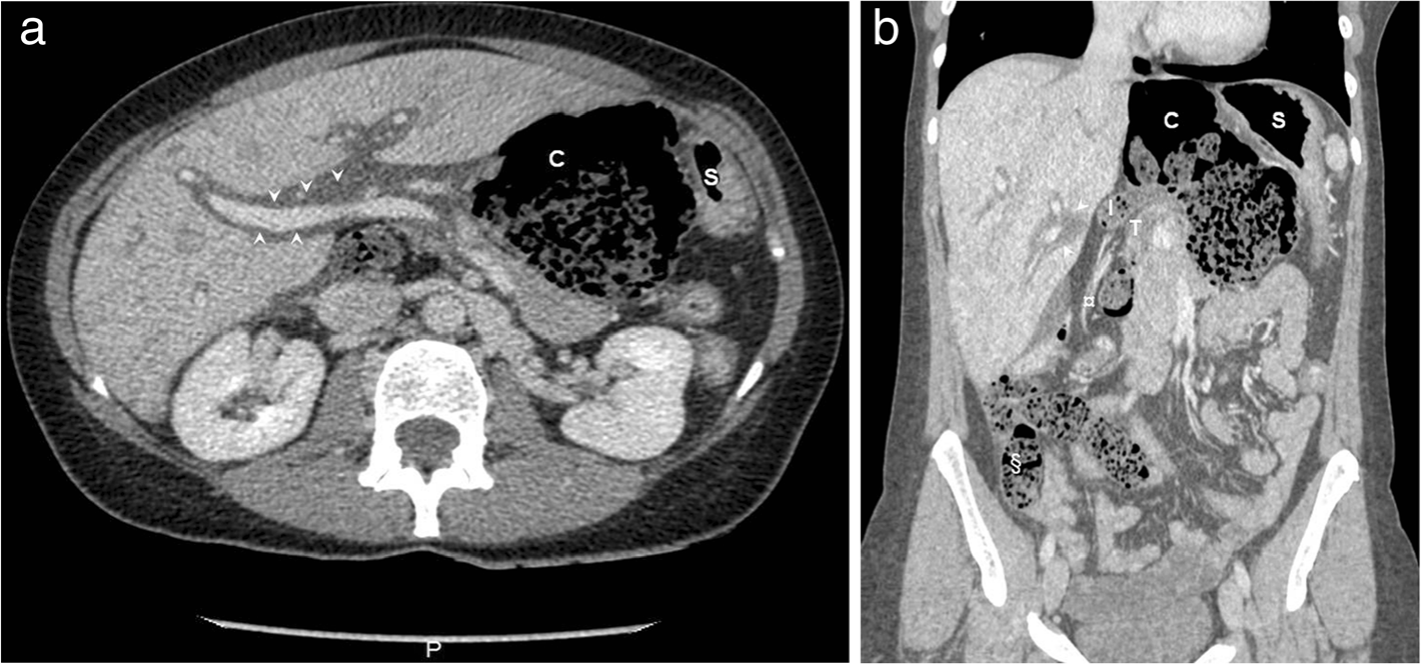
Abdominal contrast-enhanced computed tomography. a Axial section shows caecal herniation (*C*) in the lesser sac causing anterior and lateral displacement of the stomach (*S*). Edematous infiltration seen along the portal pedicles (*arrow heads*). b Coronal reconstruction demonstrates the ileocaecal pedicle (*¤*), the terminal ileum (*I*) and the transverse colon (*T*) trapped within the foramen of Winslow. Ileal loops in the *lower right quadrant* present with the “faeces sign’ (*§*) in the absence of the caecum
